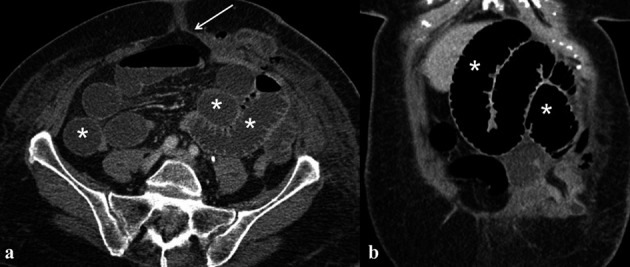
a-b: Bowel obstruction in a Patient with previous laparoscopic resection of ovarian endometrioma. a-b) axial and coronal CT images show air-fluid level in dilatated small bowel loops (white asterisks) with erniation through the surgical access (white arrow).
